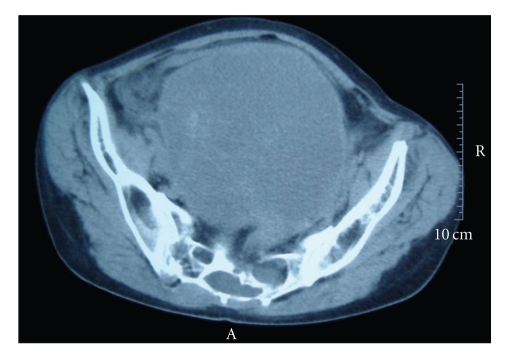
CT scanner image. Huge ovarian mass involving the anterior abdominal wall can be appreciated.
